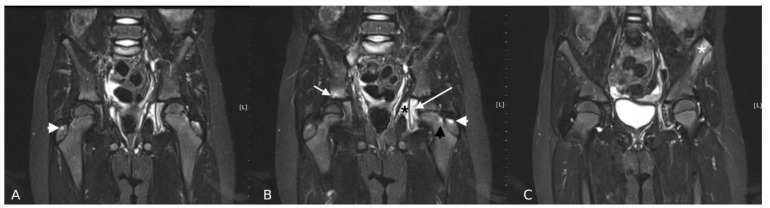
MRI of the hips in a 7-year-old girl with JIA. (A–C) Coronal T2 TIRM images. Right hip: BME in the greater Table 1. (arrowhead) and in the acetabulum (stage 1) (white short arrow). Left hip: BME in the greater trochanter (stage 1) (white arrowhead), in the acetabulum (stage 2) and triradiate cartilage (stage 1) (white long arrow) and in the femoral neck (stage 1) (black arrowhead). Obturator internus myositis (stage 1) (black asterisk). Left anterior superior iliac spine (ASIS) enthesitis (white asterisk). Right sided sacroiliitis.
